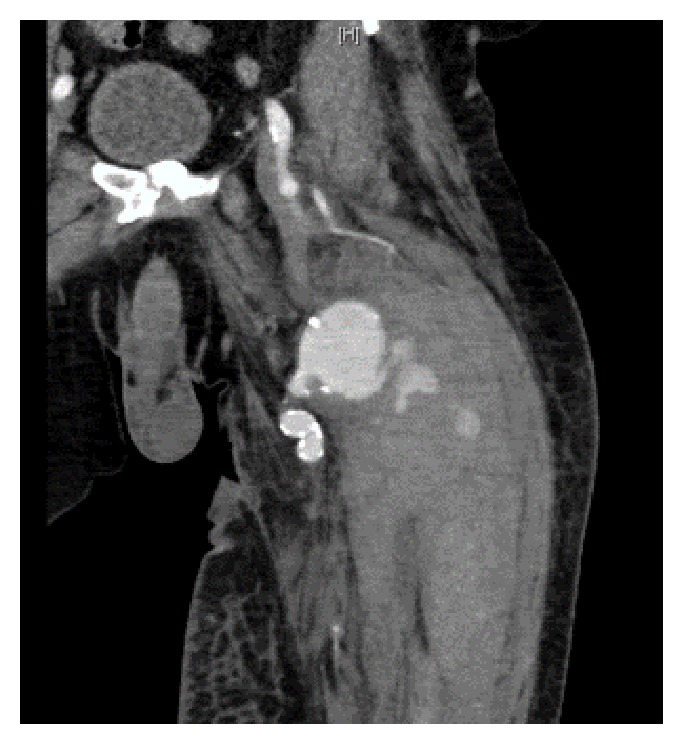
CTA of left lower extremity, coronal images showing the anastomosis pseudoaneurysm with active extravasation.
